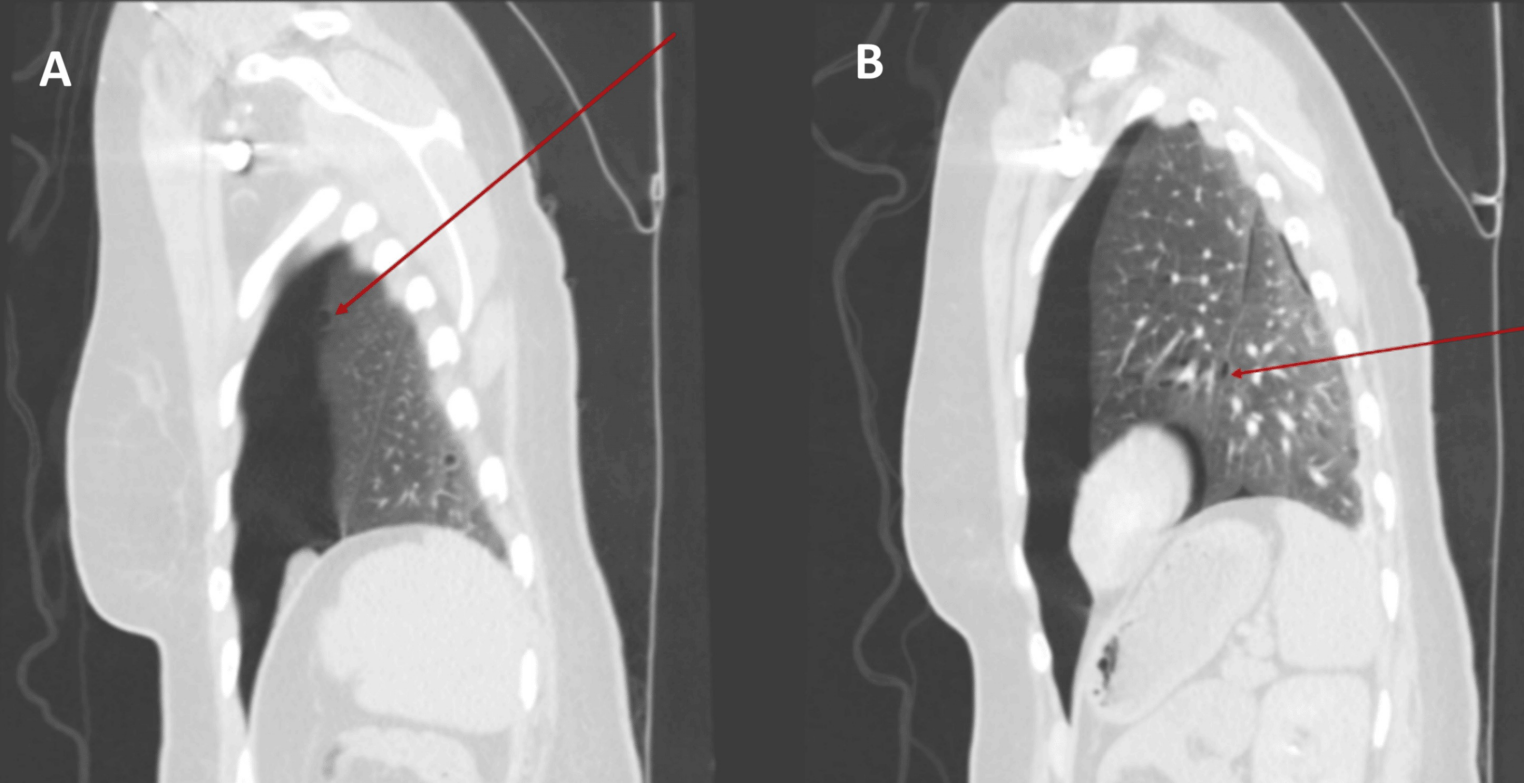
High-resolution CT chest (sagittal view) showing (A) the site of bleb rupture in the left upper lobe, and (B) additional thin-walled cystic lesions within the pulmonary parenchyma.
